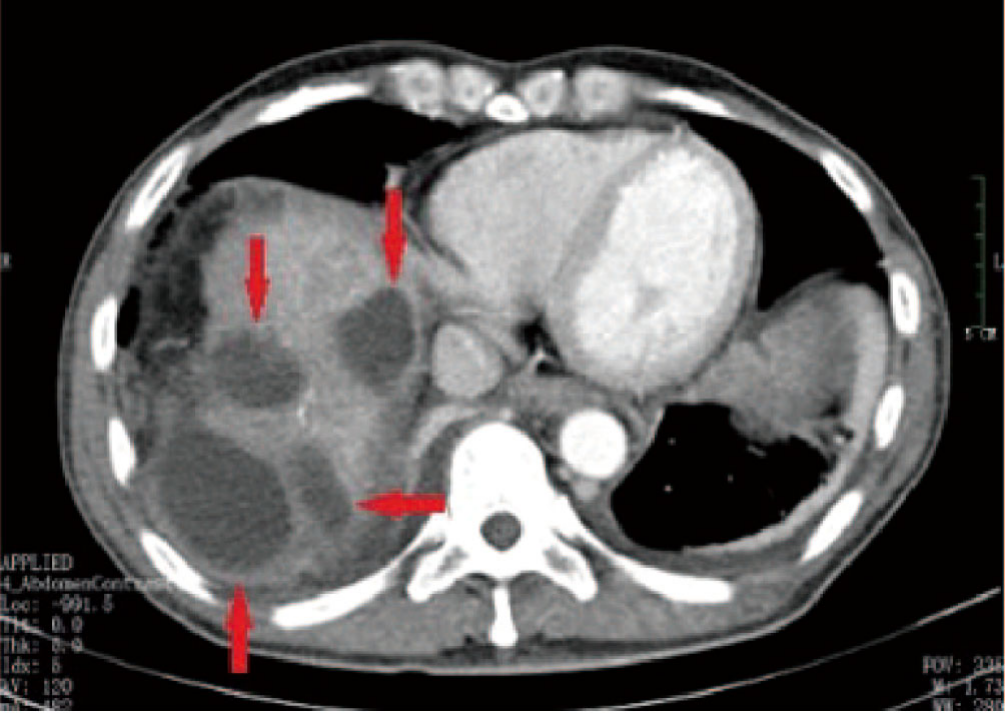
A 52-year-old male patient with HCC who received multiple DEB-TACE treatment sessions complained of abdominal pain accompanied by high a fever 4 weeks after undergoing the procedure. CT findings indicated multiple low-density shadows in the liver without obvious enhancement (red arrow). During puncture catheter drainage, we found that the drainage was a clear, yellowish biliary fluid, not liver abscess suppurative fluid, and the bacterial culture of the fluid was negative on multiple occasions.
